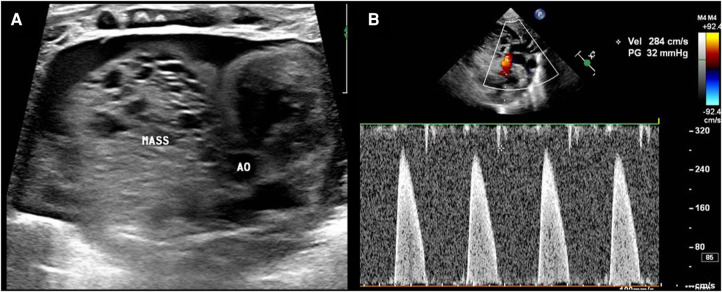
Postnatal ultrasound images. (A) The mass (MASS) is close to the ascending aorta (AO), and the boundary is not clear. (B) The mass compresses the ascending aorta, and the velocity in the ascending aorta reaches approximately 2.8 m/s.
